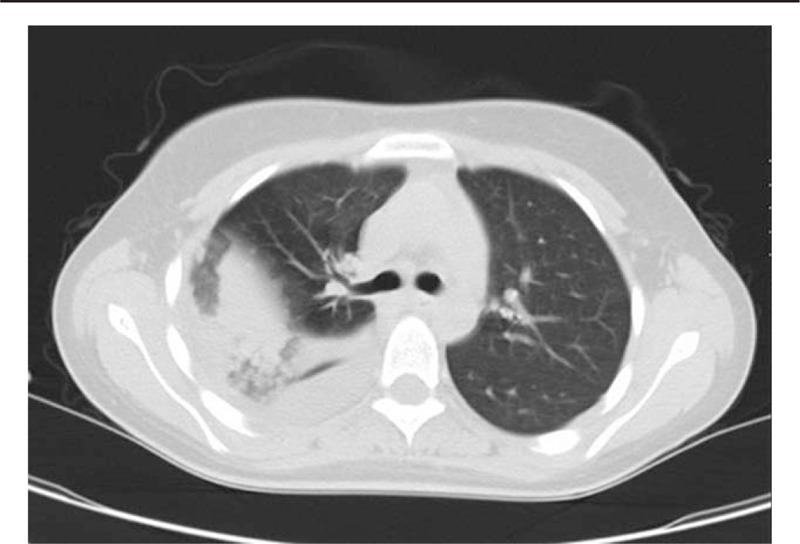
Thoracic computed tomography (CT), thoracic CT of the patient revealed lobar pneumonia.
